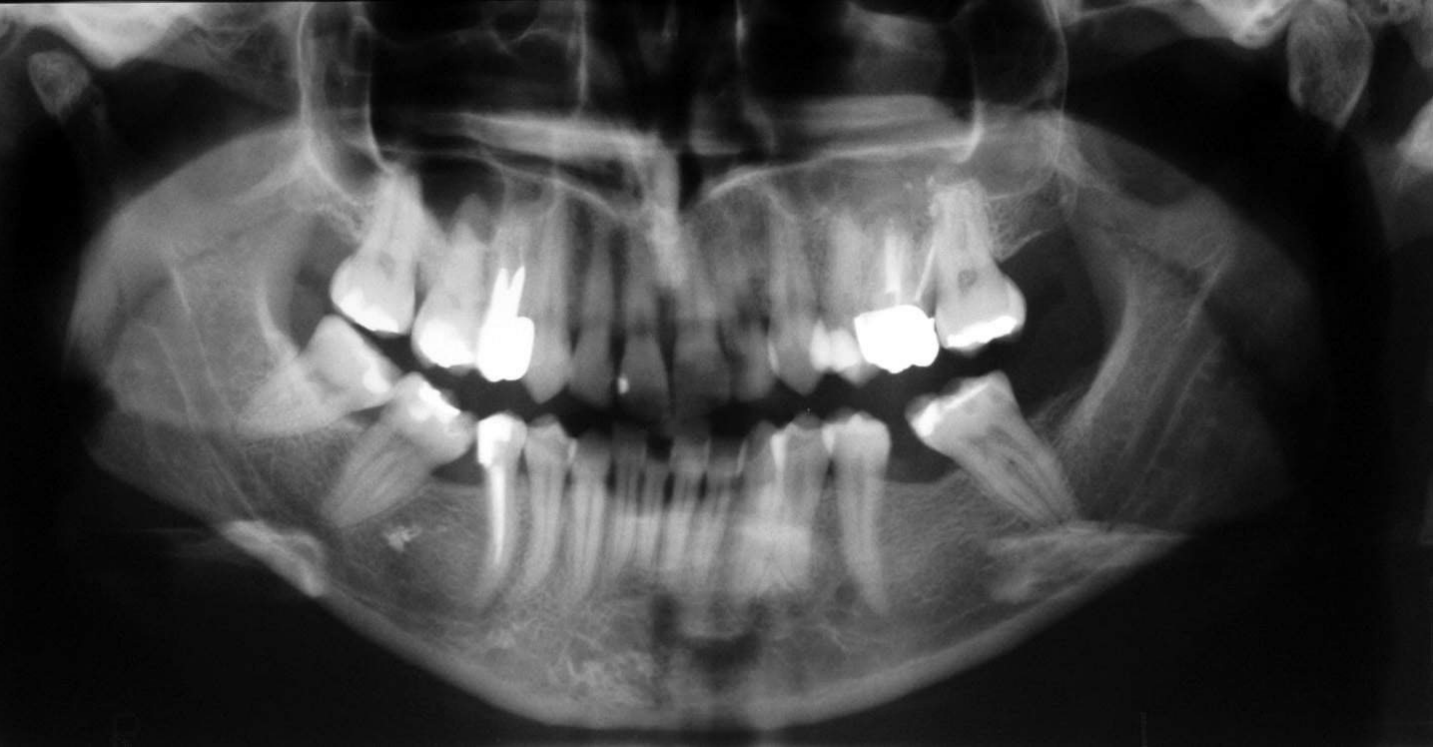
Orthopantomography showing radiopacity of the left maxillary sinus.
